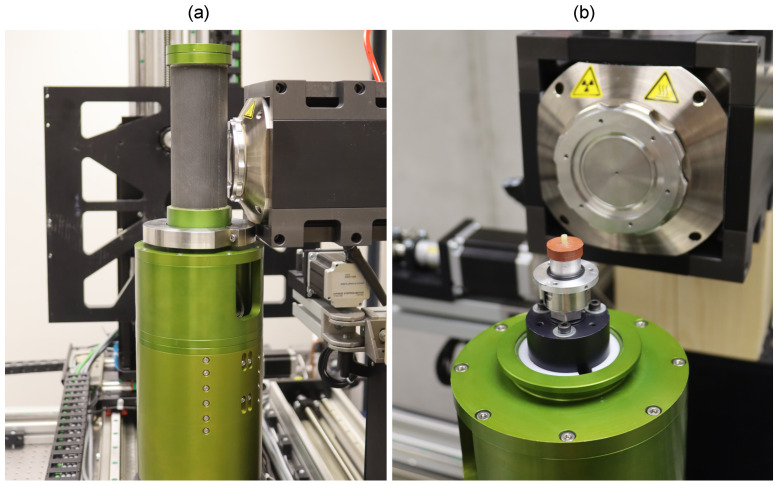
The experimental setup with the in situ loading device mounted in the X-ray scanner: (a) the scanning position for maximal magnification and (b) a detailed view on the open device with the sample placed for testing.
